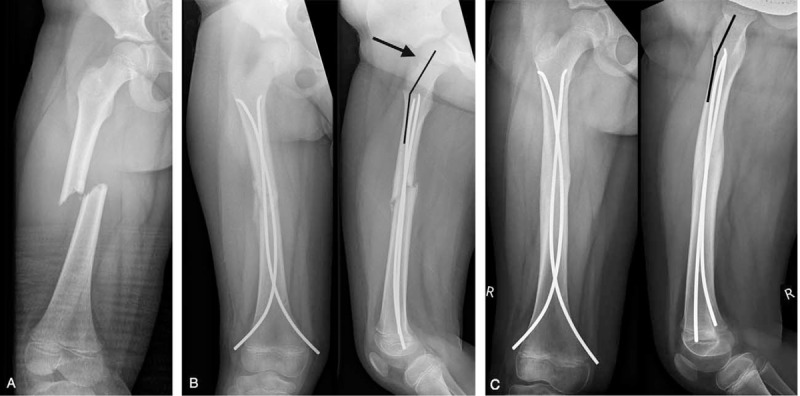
A 5-year-old girl sustained a short oblique fracture of the femur when injured in a pedestrian-motor vehicle collision. (A) The radiographs obtained 4 weeks after the injury showed moderate retrotorsion of the femoral neck. This external rotational malalignment should not have been accepted (B). Union of the fracture was confirmed by radiographs after 5 months, and there was minimal retrotorsion of the femoral neck (indicated by the angle) in the lateral view indicative of minimal external rotational malalignment (C).
